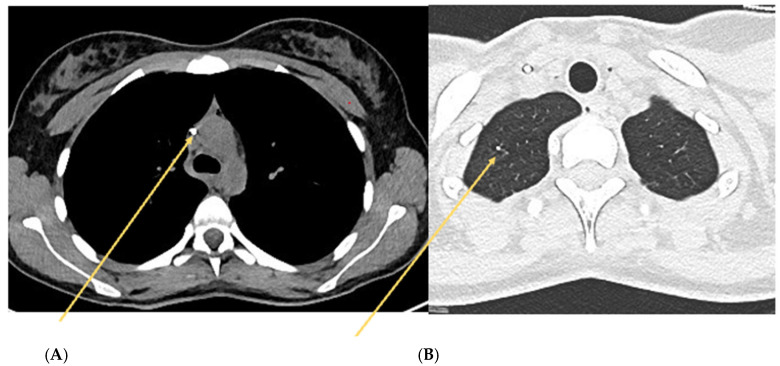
Chest CT findings demonstrating calcifications in the intrathoracic paravascular lymph nodes (A) and a calcification in the upper lobe of the right lung (B).
